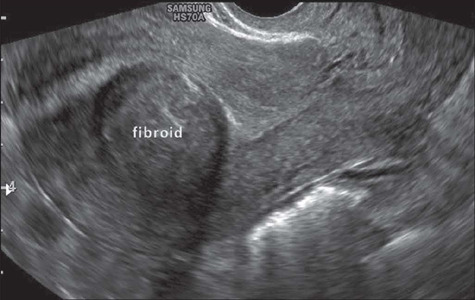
Transvaginal ultrasound image, in a longitudinal view, showing a submucosal (FIGO
